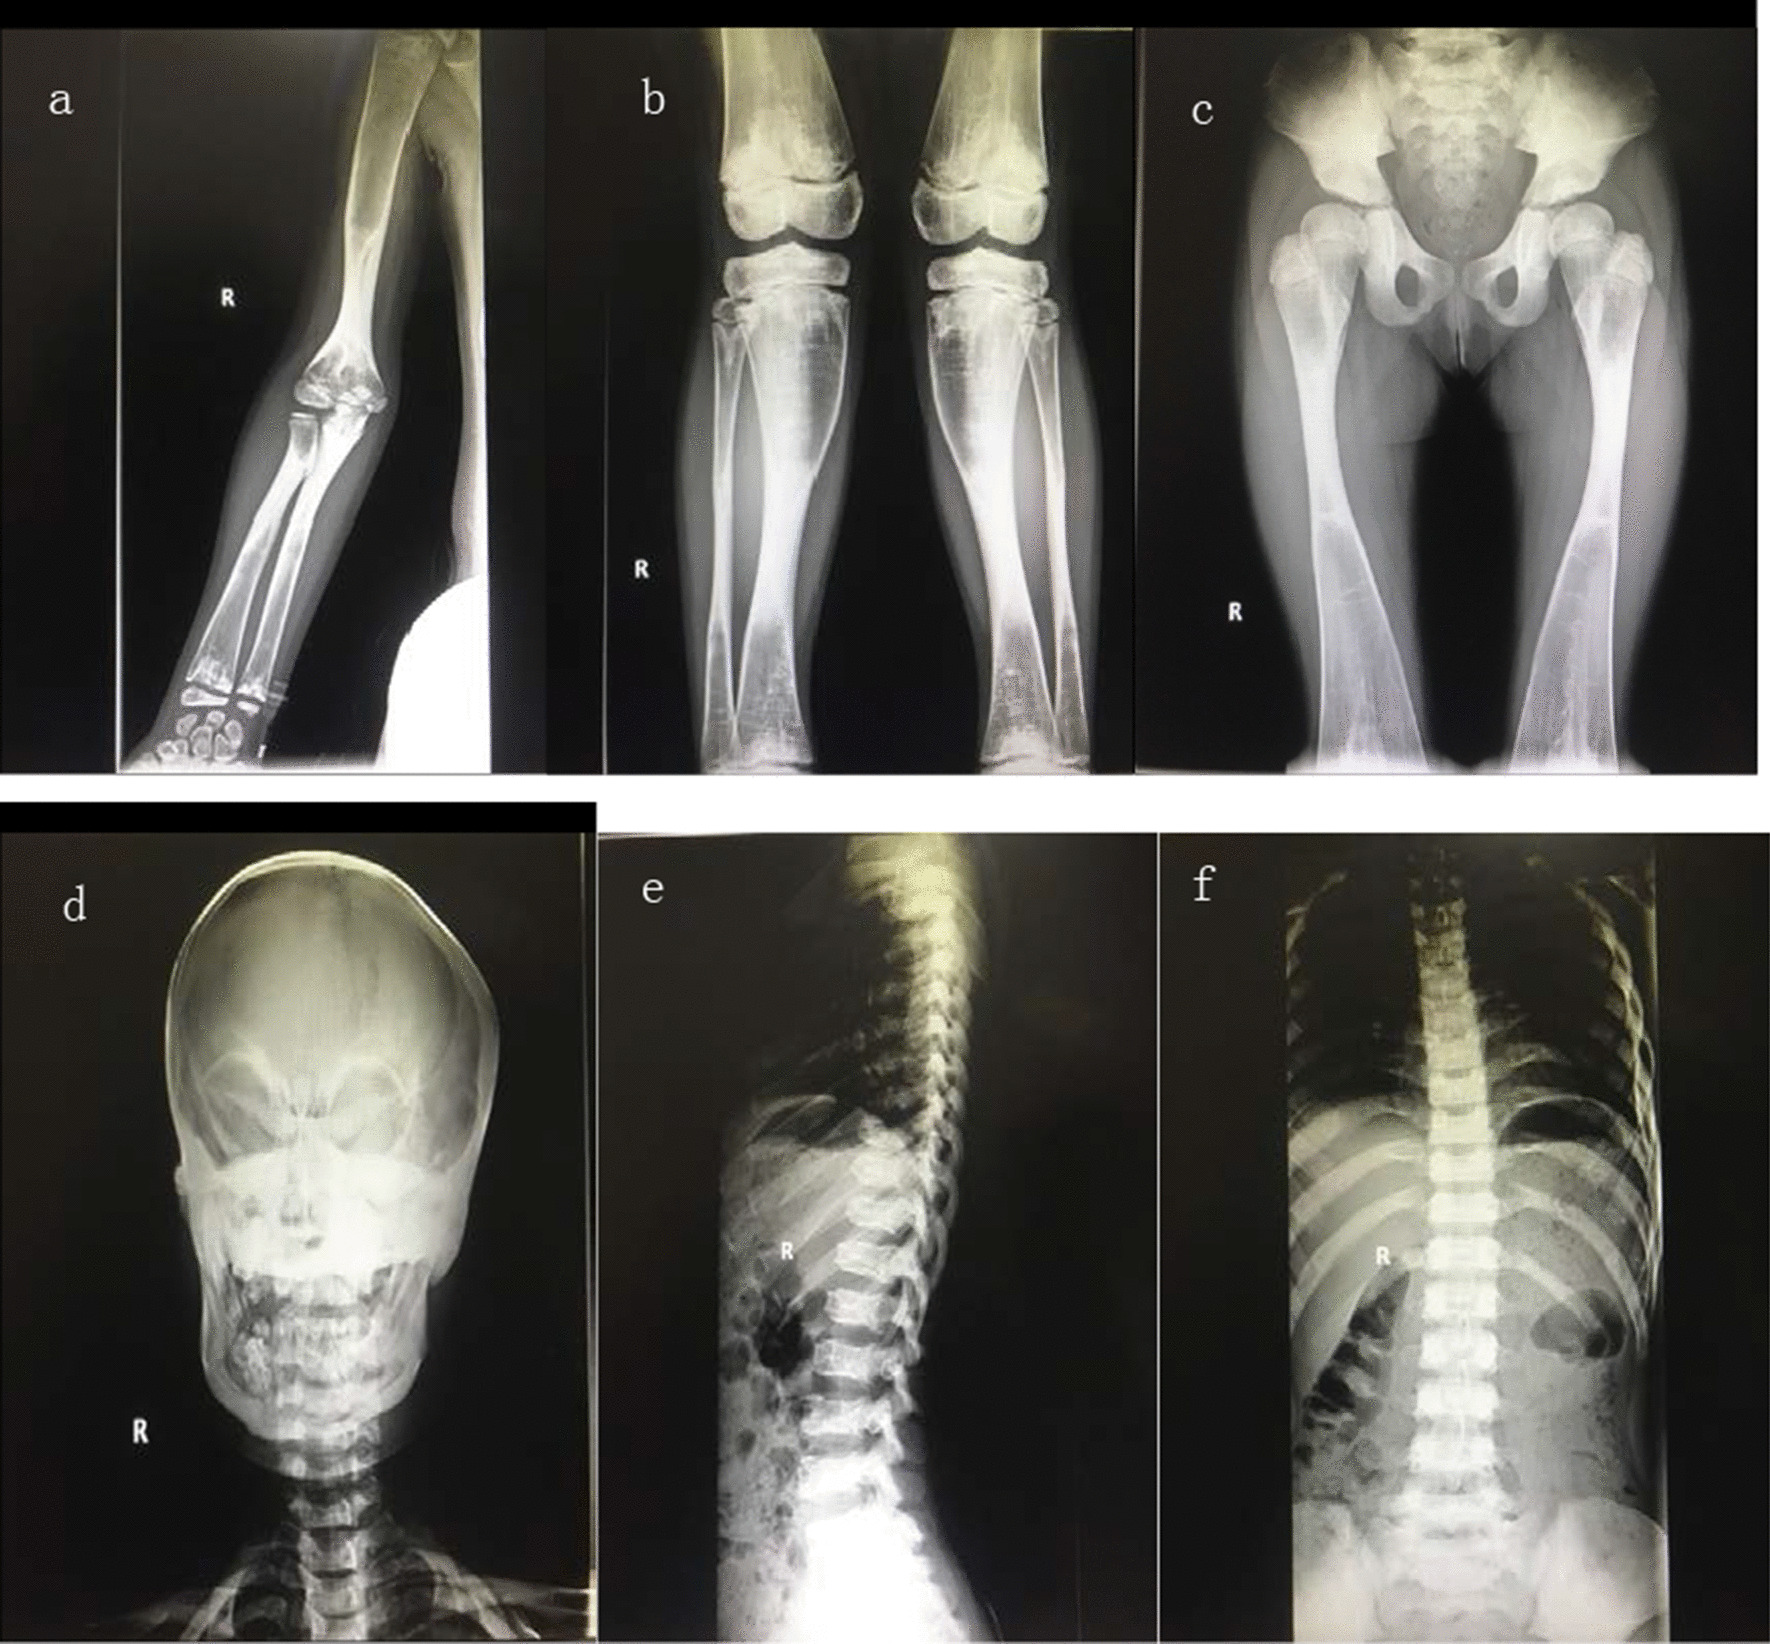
a–c The structure of limb bones is deformed. Extreme metaphyseal flaring, reduced bone vascularity and dense bone structure is found. d Skull radiograph showing typical wolf carnival appearance. e, f Front-Lateral Chest radiograph showing diffuse sclerotic vertebra and costochondral junction widening
